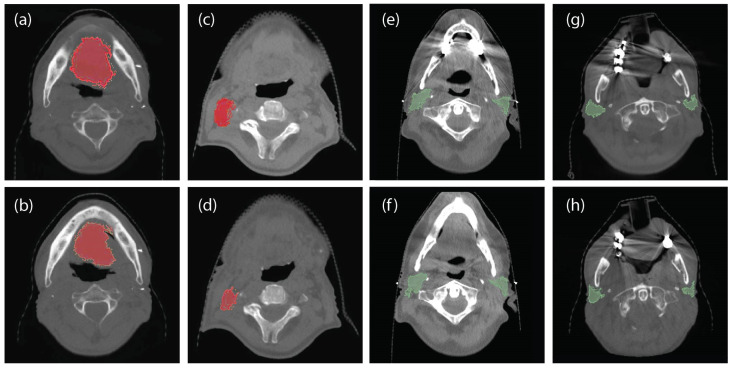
CBCT scans for patients at baseline (week 0) and at the end of the RT treatment sessions with significant and non-significant changes for the CTV tumor region (red mask) and the PG tumor region (green mask). Specifically: (a) CTV ROI of patient #3 at baseline; (b) CTV ROI of patient #3 at the end of the RT treatment sessions (non-significant changes); (c) CTV ROI of patient #14 at baseline; (d) CTV ROI of patient #14 at the end of the RT treatment sessions (significant changes); (e) PG ROI of patient #3 at baseline; (f) PG ROI of patient #3 at the end of the RT treatment sessions (non-significant changes); (g) PG ROI of patient #18 at baseline; (h) PG ROI of patient #18 at the end of the RT treatment sessions (significant changes).
